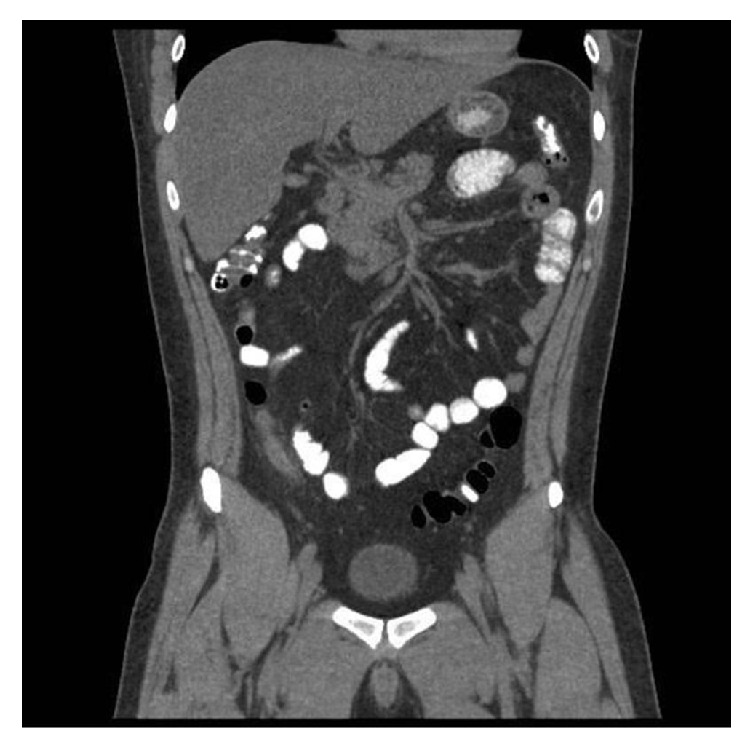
Coronal section of abdominal CT scan with contrast demonstrating swollen appendix with 9.8 mm diameter, no appendicolith present.
